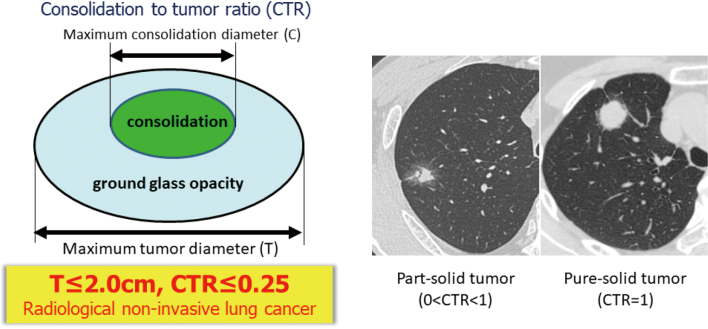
Definition of the consolidation to tumor ratio, and the typical findings of part-solid tumor and pure-solid tumor based on thin-section computed tomography^1)^.
